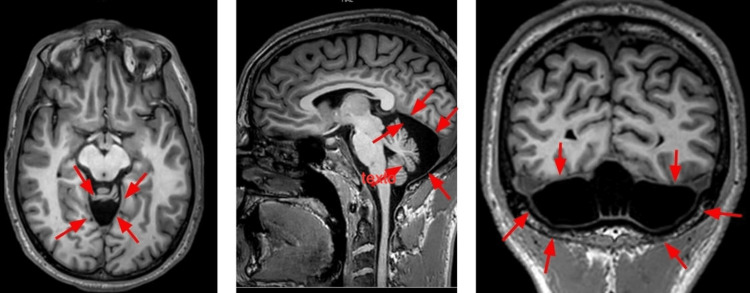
One month later, cerebral MRI demonstrated a mega cisterna magna (red arrows).
